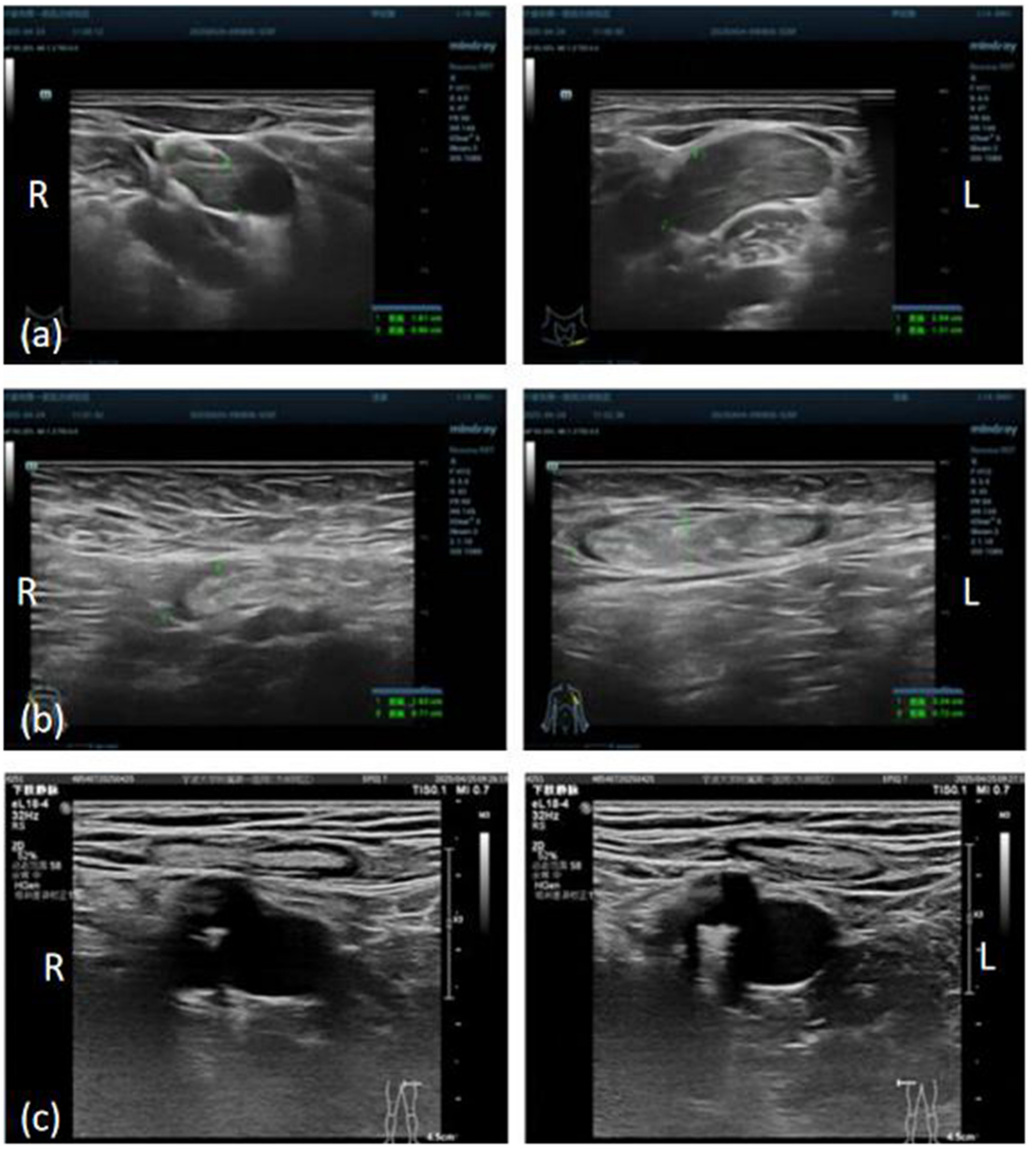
Ultrasound of superficial lymph nodes demonstrated multiple enlarged lymph nodes in the bilateral supraclavicular (a), axillary (b), and inguinal (c) regions.
